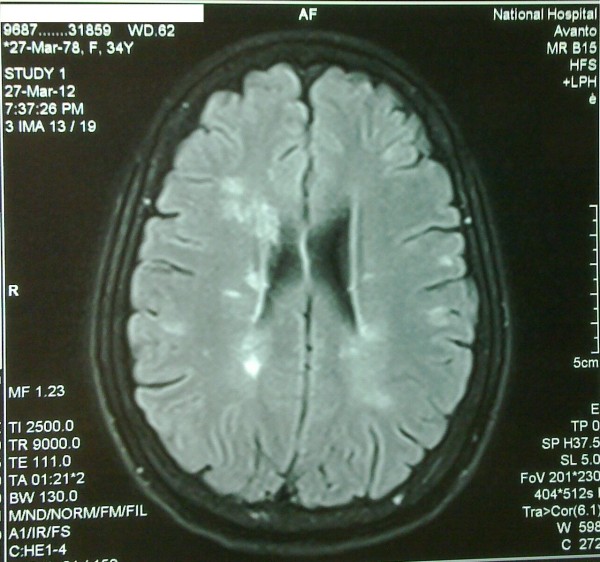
Close up image of T2WI MRI Brain image of multiple periventricular hyperintense lesions.
